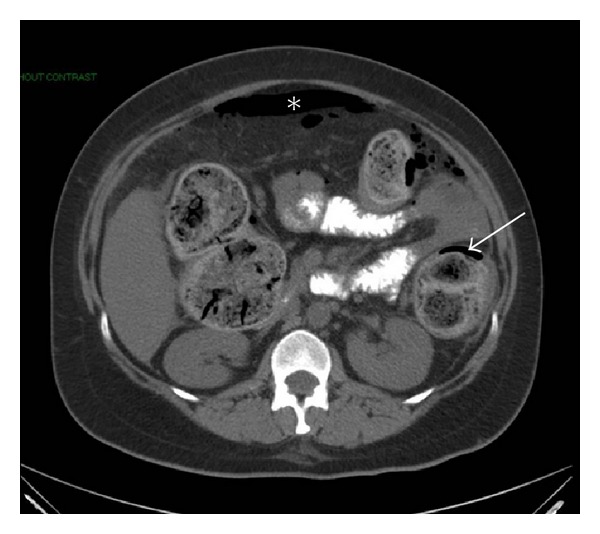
CT scan of the abdomen with free air (star) and air in bowel wall (arrow).
